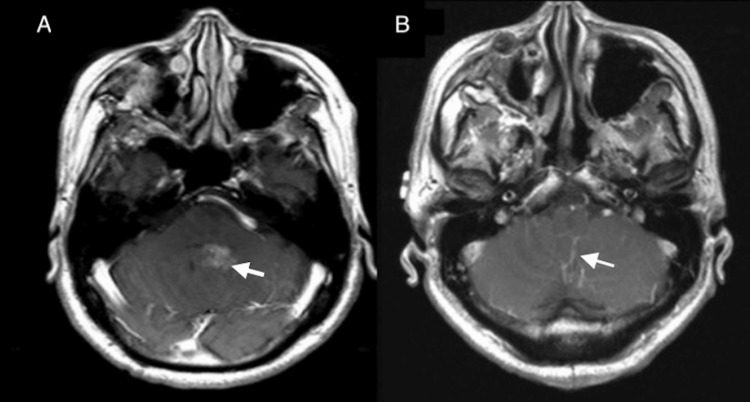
Ten-year follow-up showing mass in the fourth ventricle A: Preoperative MRI, ten years after initial surgery, presents an enhancing mass in the fourth ventricle. B: Postoperative axial postcontrast T1-weighted MRI after suboccipital craniotomy demonstrates gross total resection of the mass in the fourth ventricle.
